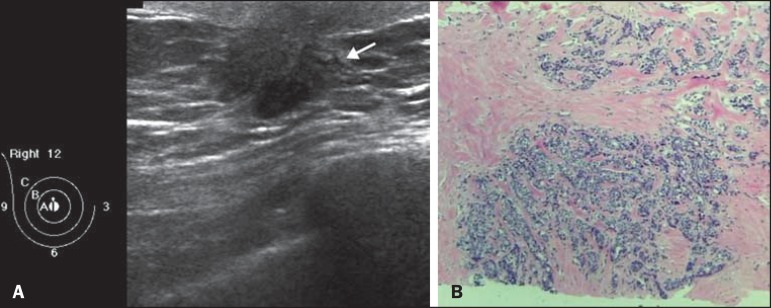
A: Ultrasound showing a solid, hypoechoic, irregular spiculated
